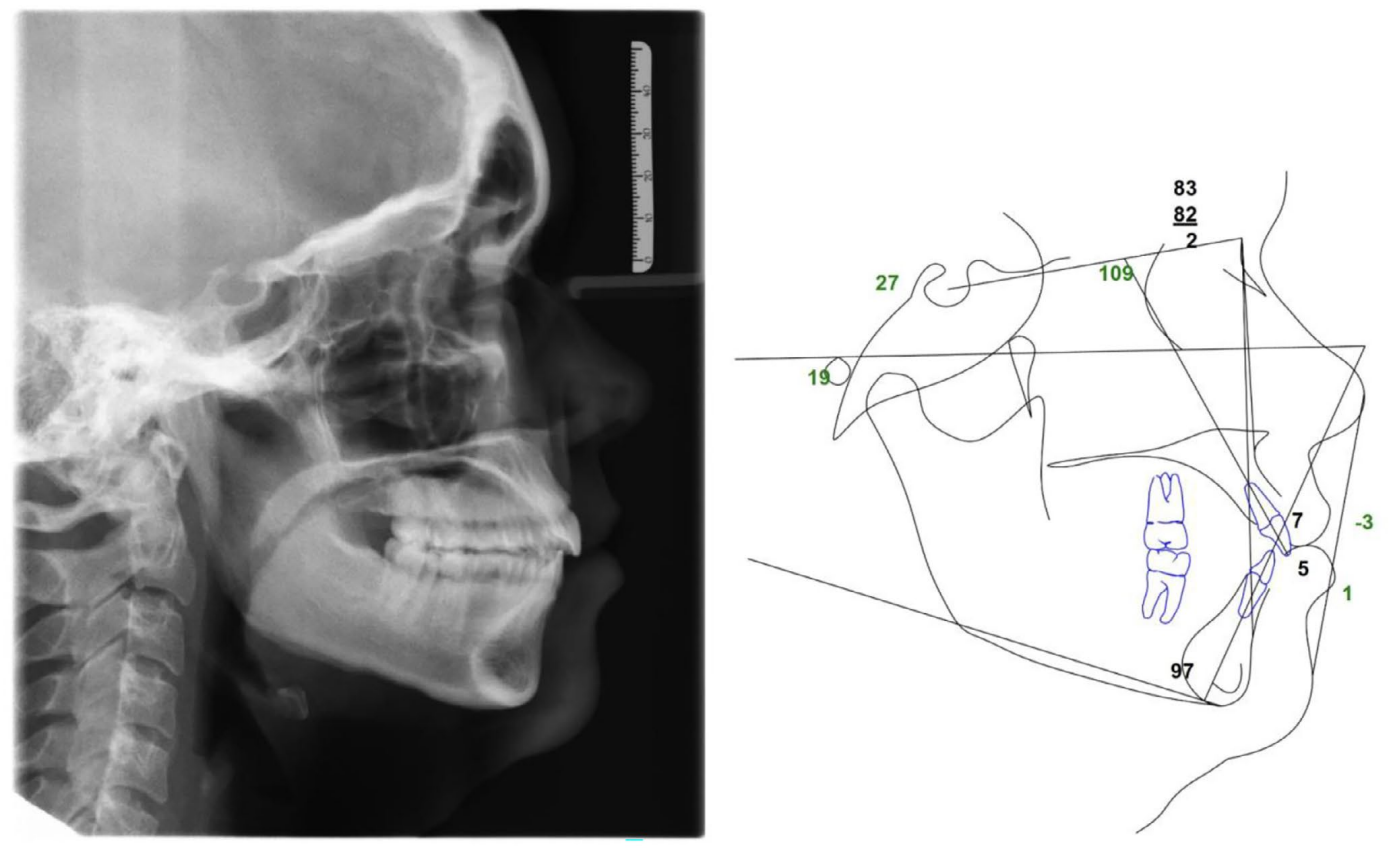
Post‐treatment cephalometric radiograph and tracing show closed anterior open bite and class I occlusion.
